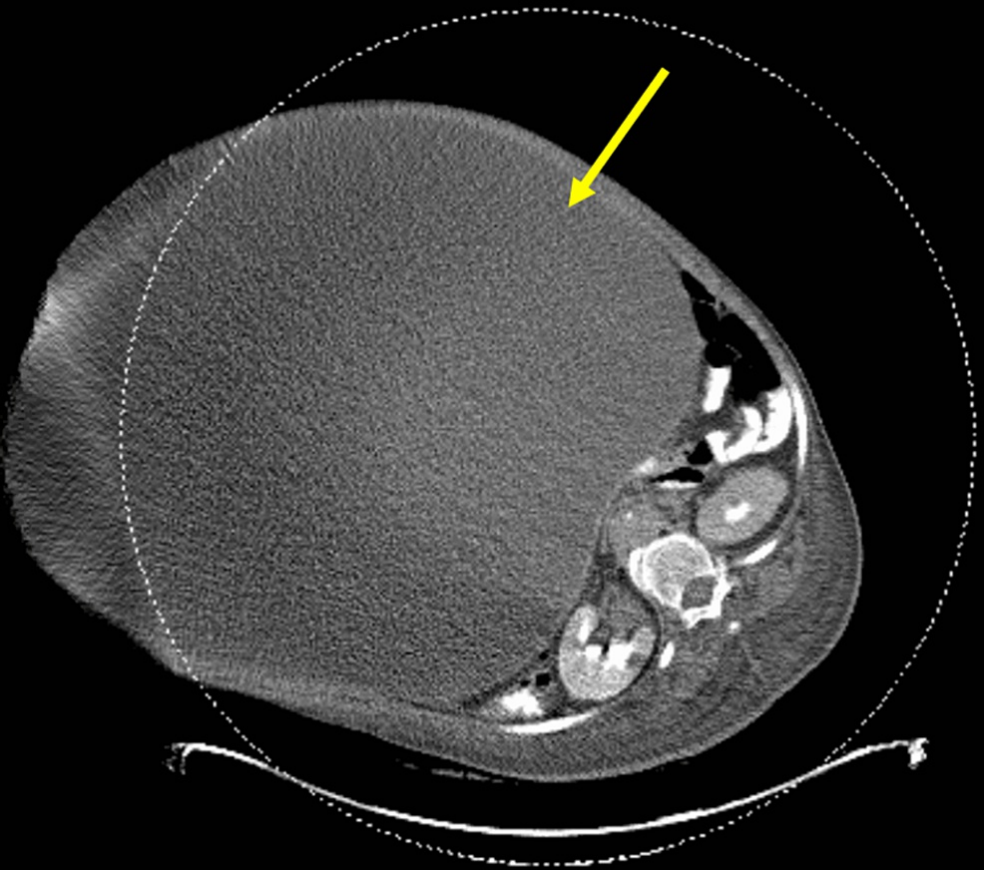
Computed tomography showing the large intra-abdominal mass
